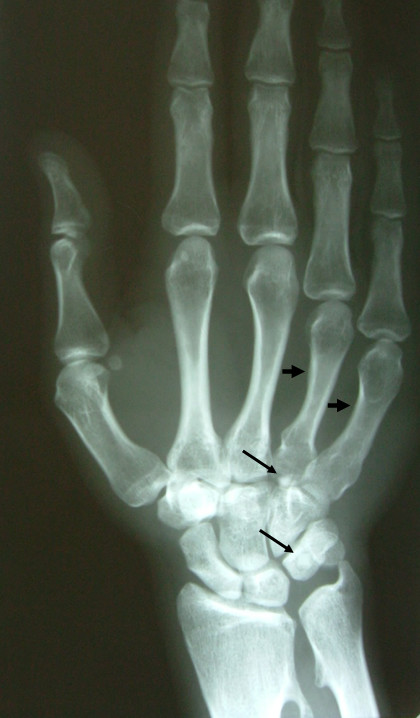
AP radiograph of the hand showing the presence of short 4^th ^and 5^th ^metacarpals (short arrows) and the presence of two sesamoids anterior to the triquetrum and hamate (long arrows).
